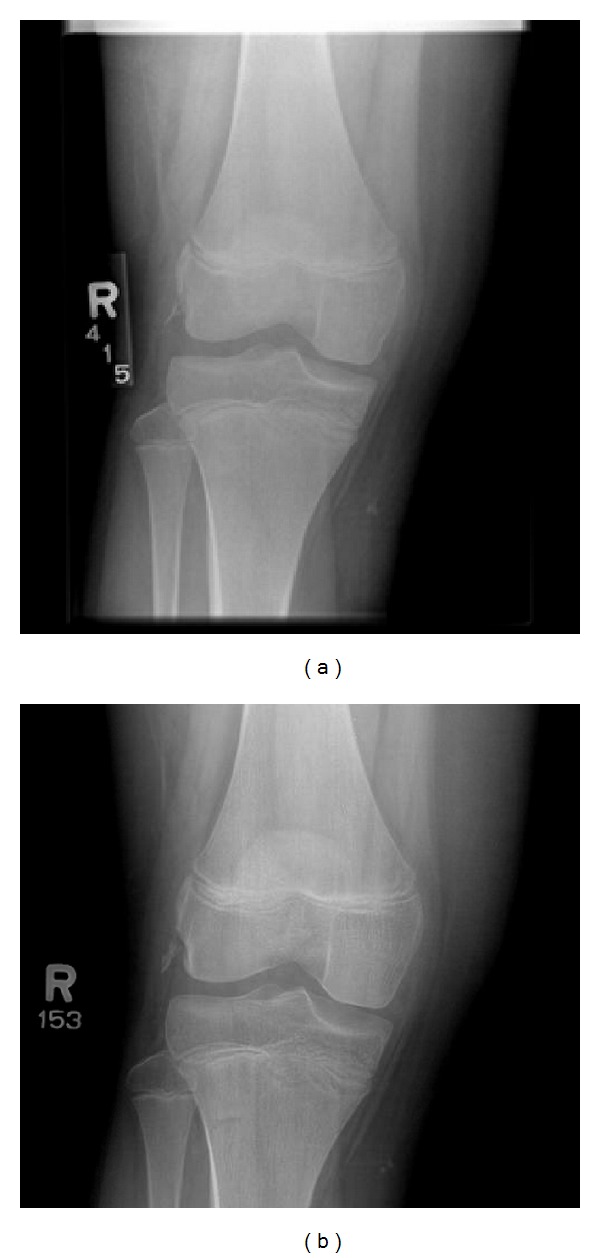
(a) AP radiograph showing avulsion fracture in the area of the popliteus tendon. (b) 6-week follow-up radiograph showing persistent fragment displacement.
